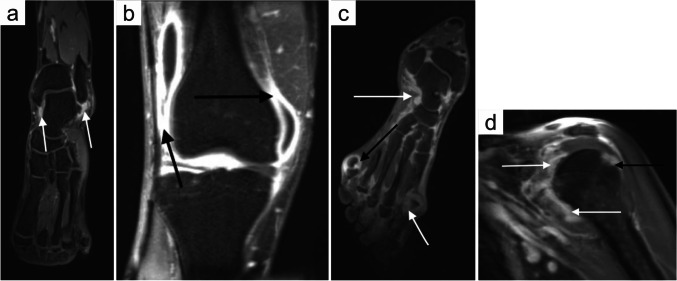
Post-contrast T1w VIBE Dixon water-only images from an abbreviated “multi-joint” MRI protocol (pseudowbMRI) in two patients with rheumatoid arthritis (a–b and c–d). a Oblique axial image of the ankle showing synovitis of the tibiotalar joint (white arrows); b Coronal image of the knee showing marked synovitis (black arrows) and joint effusion; c Oblique axial image of the foot showing synovitis (white arrows) and erosion (black arrows). d Coronal image of the shoulder showing extensive synovitis (white arrow) and erosion of the humeral head (black arrow) (reproduced from Freeston et al., 2024 [67], originally licensed under CC BY 4.0)
